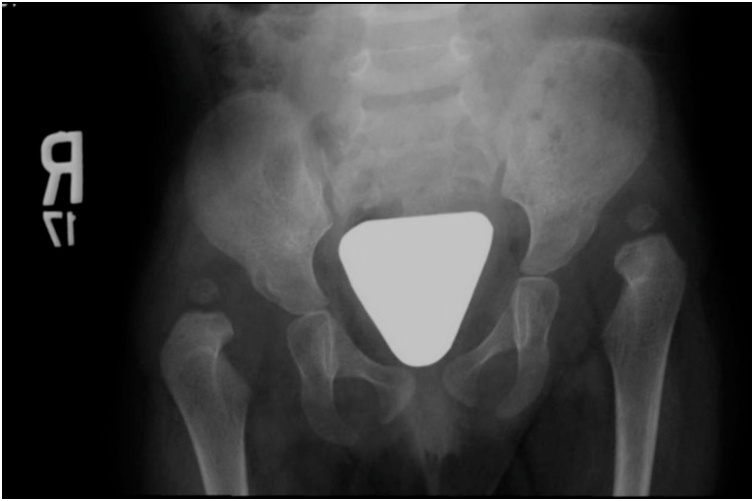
Pelvis AP x-ray showing bilateral hips dislocated DDH at age of 2 years, Right Tonnis 3, left Tonnis 4.
